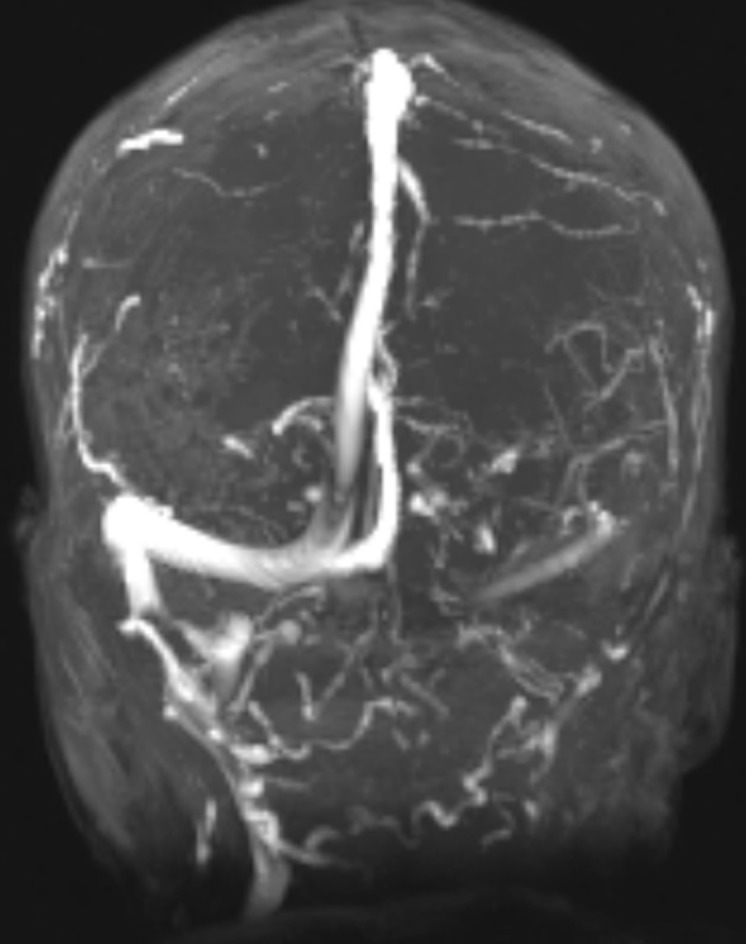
brain magnetic resonance imaging (MRI) in time-of-flight (TOF) sequence showing no visualization of the left sigmoid sinus
